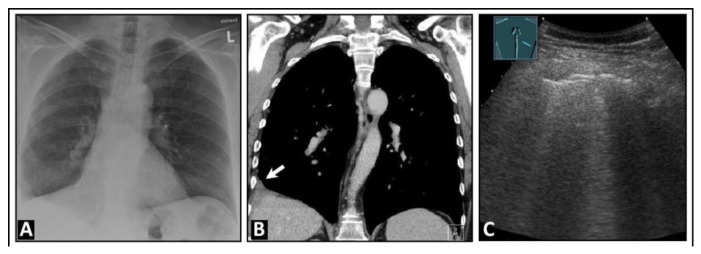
A patient experiencing pain in the right thoracic region and an obscured right diaphragmatic costal angle on (A) chest X-ray and (B) chest computed tomography (indicated by arrow). (C) On lung ultrasound, multiple B-lines (arrows) are seen in this area, accompanied by a rough, interrupted pleural reflex line (arrowheads), consistent with a focal interstitial pattern, most likely due to scarring.
